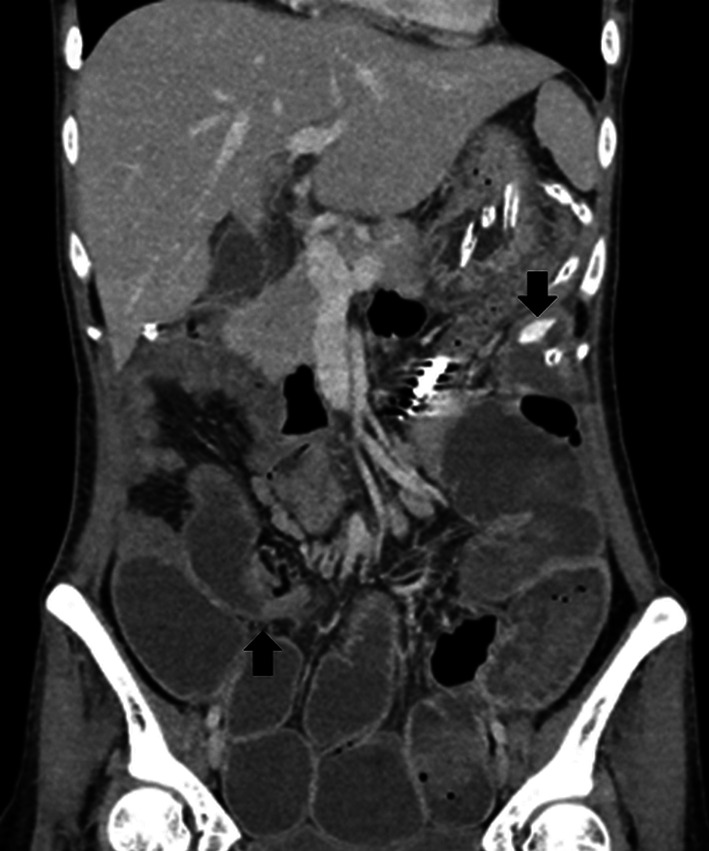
Computed tomography image taken before reoperation. Computed tomography image before reoperation showed extravascular leakage (upper arrow) and caliber change (lower arrow)
